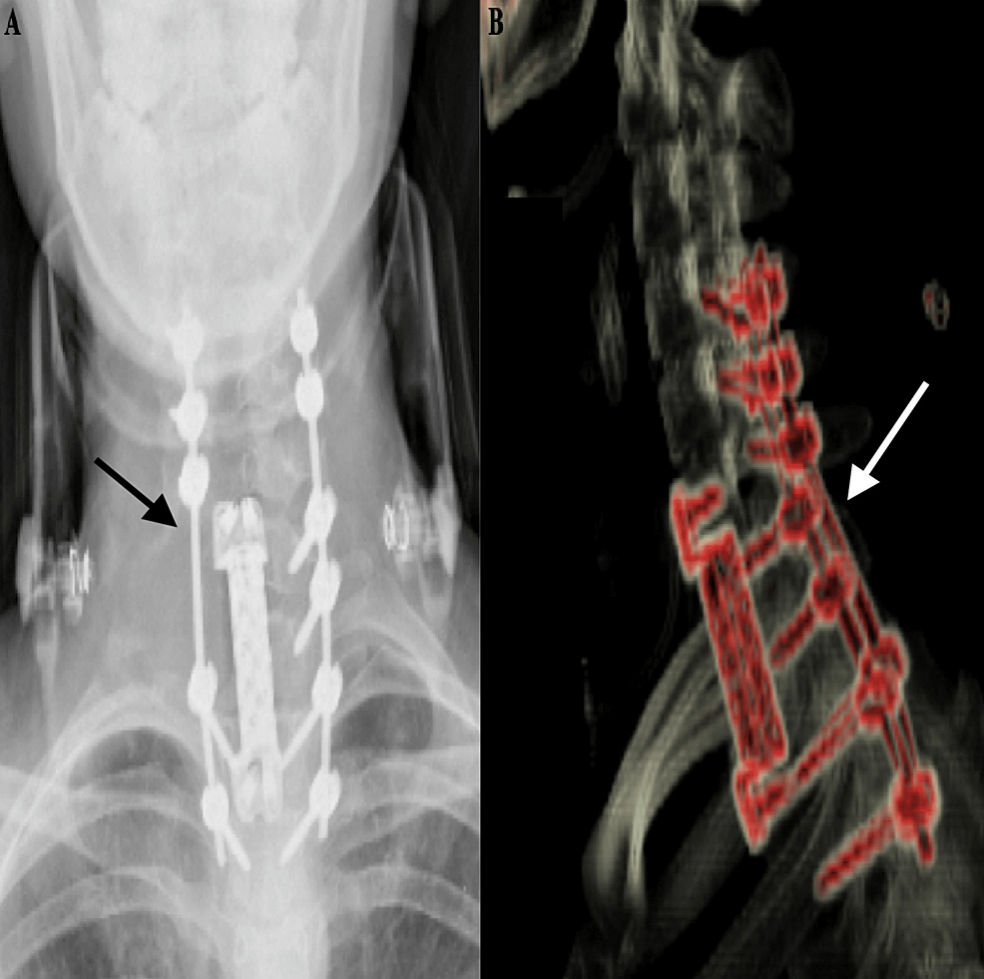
(A) Postoperative anteroposterior radiograph. (B) Postoperative three-dimensional computed tomography image.
